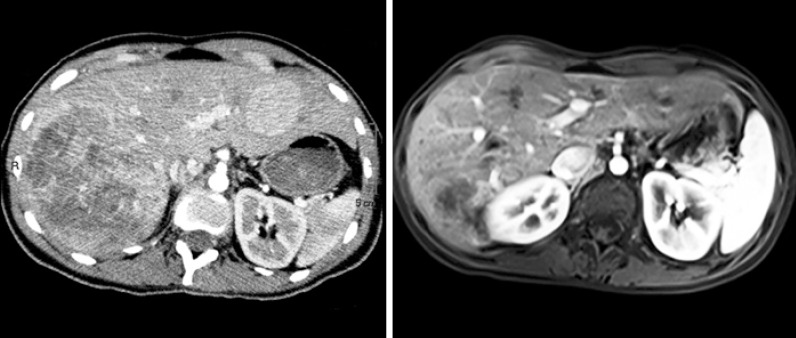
Tomography of the abdomen before embolization and control magnetic resonance imaging one year later. A large hypervascular lesion is observed in the right liver lobe with a heterogeneous aspect suggestive of local hematoma, hypervascular lesions in the left liver lobe, and free fluid in the peritoneal cavity suggestive of abdominal hemorrhage. The magnetic resonance imaging exam one year later showed a significant reduction of the embolized lesions and the absence of new lesions
